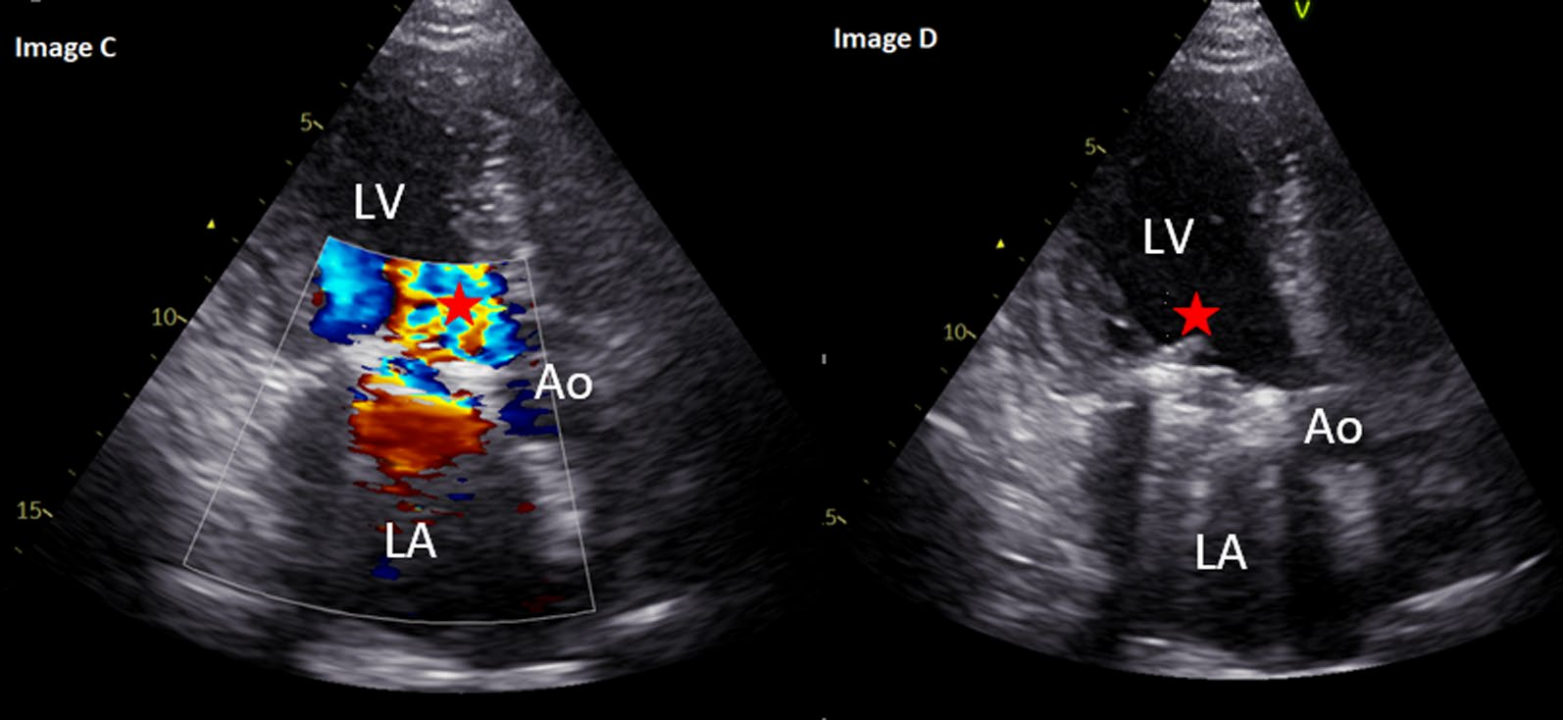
Images C and D: A 54-year-old female with a mechanical mitral valve replacement in 2012 for severe mitral stenosis secondary to rheumatic fever. In April 2020, the patient presented acutely with fever and night sweats. Blood cultures were positive for Staphylococcus aureus. Transthoracic echocardiography identified a stable in-situ mechanical mitral valve with good occluder mobility. There was turbulent forward flow (* in image C) and significantly elevated transvalvular mean gradient of 15 mmHg (documented as 3.3 mmHg on transthoracic echocardiography 13 months prior). There was a linear mobile mass (* in image D) on the left ventricular size of the mechanical valve replacement which was not visible on previous imaging. There was a high suspicion of infective endocarditis which was confirmed on a subsequent transesophageal echocardiography. The patient was commenced on antibiotic therapy, re-do mitral valve replacement was undertaken 16 weeks later, after which the patient made a good and uneventful recovery. At last follow-up, there was a stable in-situ mechanical mitral valve replacement, mean gradient: 3.4 mmHg, normal left ventricular size and systolic function, biplane ejection fraction: 59%. The patient was asymptomatic without any reduction in exercise tolerance. The patient remains on 12 monthly follow-up. LV: left ventricle, LA: left atrium, Ao: aorta
